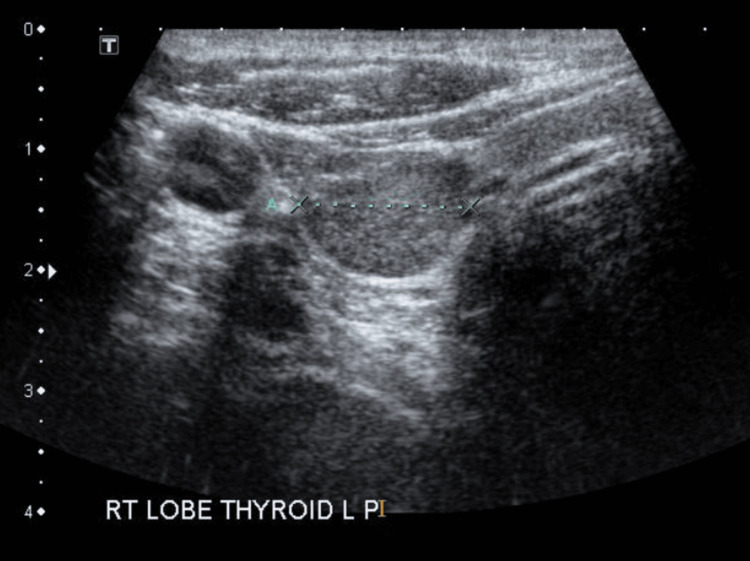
USG of the neck confirmed a right inferior parathyroid adenoma. Parathyroid adenomas on USG are seen as oval or bean-shaped, well-defined and hypoechoic masses located posterior to the thyroid gland. They are highly vascular on Doppler imaging.
